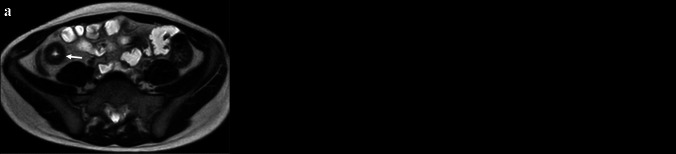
A 12-year-old male with Crohn’s disease. a Axial T2-weighted single-shot fast spin-echo (SSFSE) MR image shows ileal wall thickening (*arrow*). b Axial SSFSE through the level of the root of the small bowel mesentery shows the superior mesenteric artery and vein in cross-section (*arrows*). This anatomic level was used for phase-contrast imaging. c Axial velocity-encoded phase-contrast MRI image shows signal in the superior mesenteric artery and vein (*long arrows*) as well as in the abdominal aorta and inferior vena cava (*short arrows*). Blood flowing inferiorly is hypointense, while blood flowing superiorly is hyperintense
